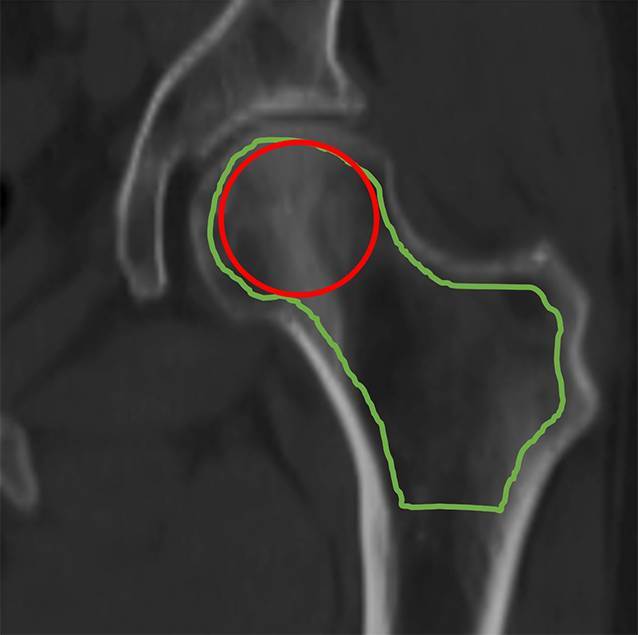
„Region of interest“ (ROI) im prox. Femur: kreisrunde ROI im Caput femoris (*rote Markierung*) sowie irreguläre Flächen-ROI (*grüne Markierung*) über die gesamte prox. Femurregion im koronar reformierten CT-Schnittbild
